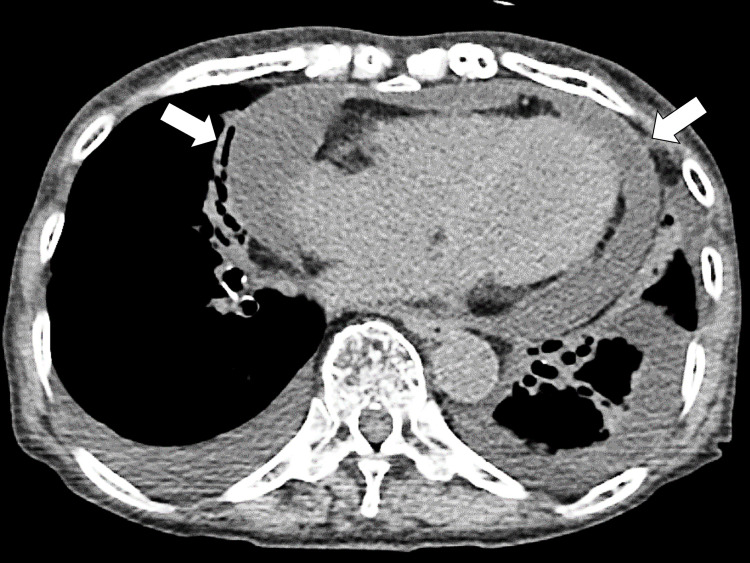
Pericardial effusion on the initial CT (arrows) CT: computed tomography
